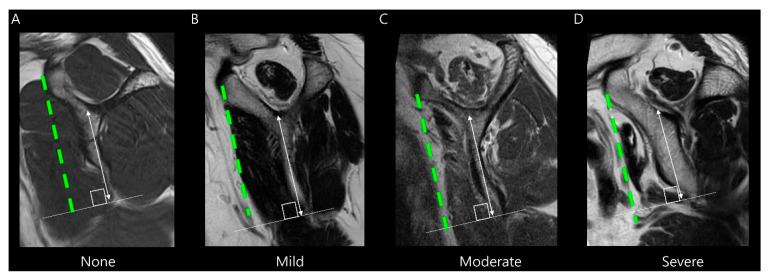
Evaluation of subscapularis muscle atrophy on T2-weighted oblique sagittal images. The grade is determined by the amount of muscle above or below a virtual line (green dashed line) drawn from the superior border of the coracoid process to the inferior margin of the subscapularis muscle at the level of the scapular inferior tip (solid line), parallel to the long axis of the scapular body (double-headed arrow). (A) None, (B) Mild atrophy, (C) Moderate atrophy, and (D) Severe atrophy.
